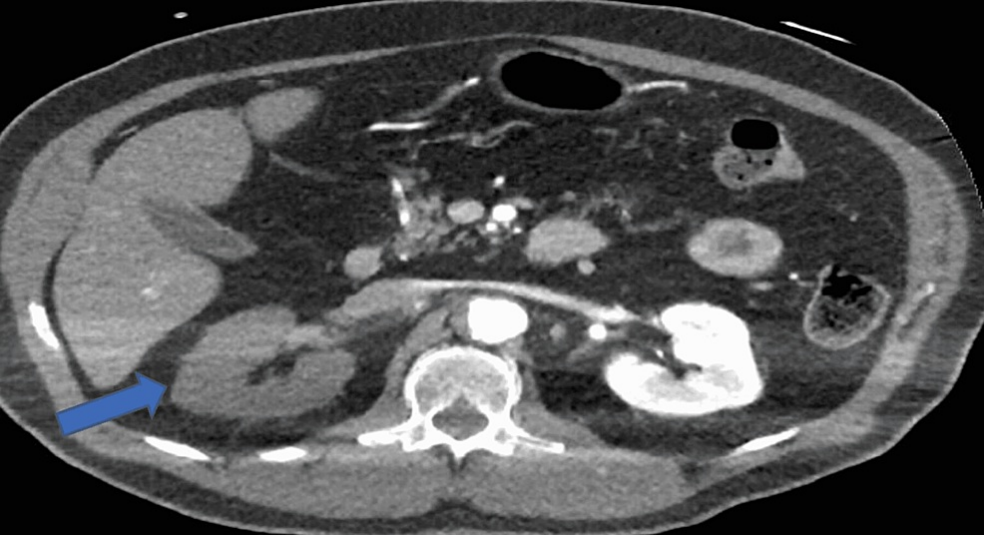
Computed tomography pulmonary angiogram showing right-sided renal malperfusion as shown by the pointed arrow
